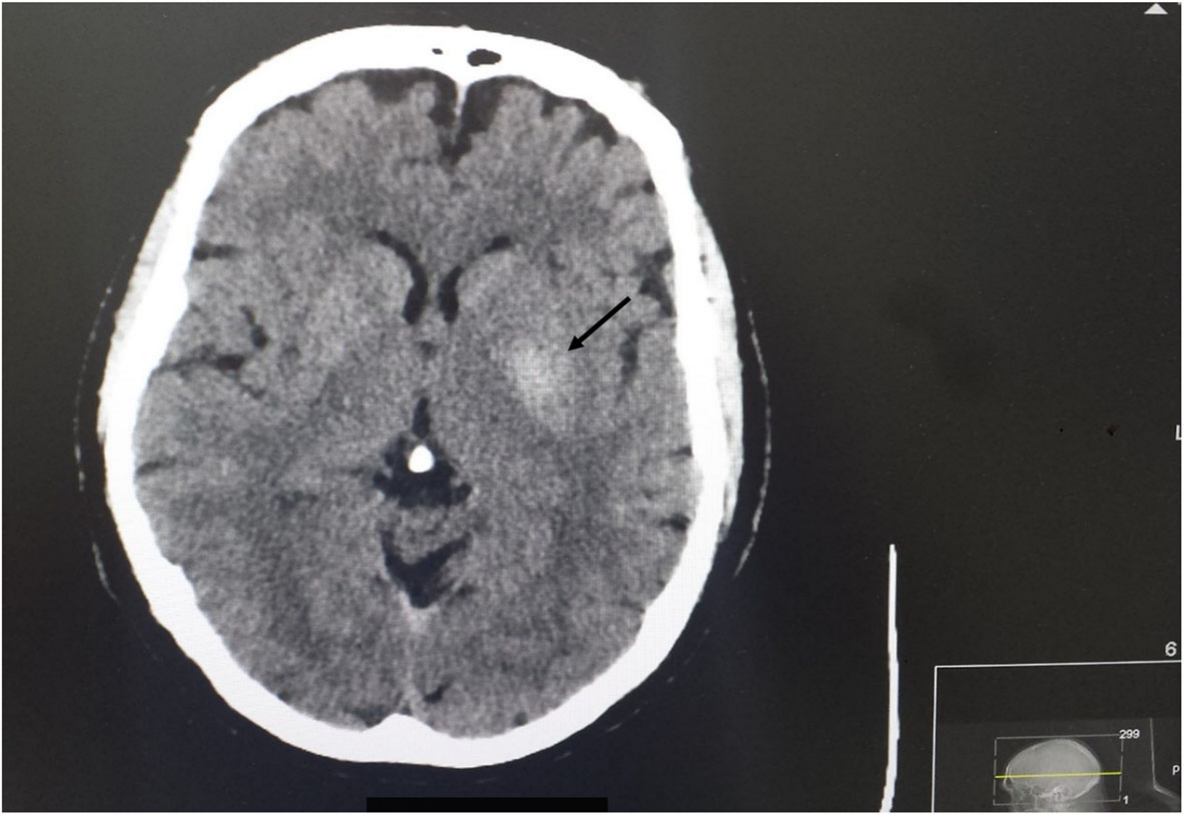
Computed tomography scan. Arrow: tumor
